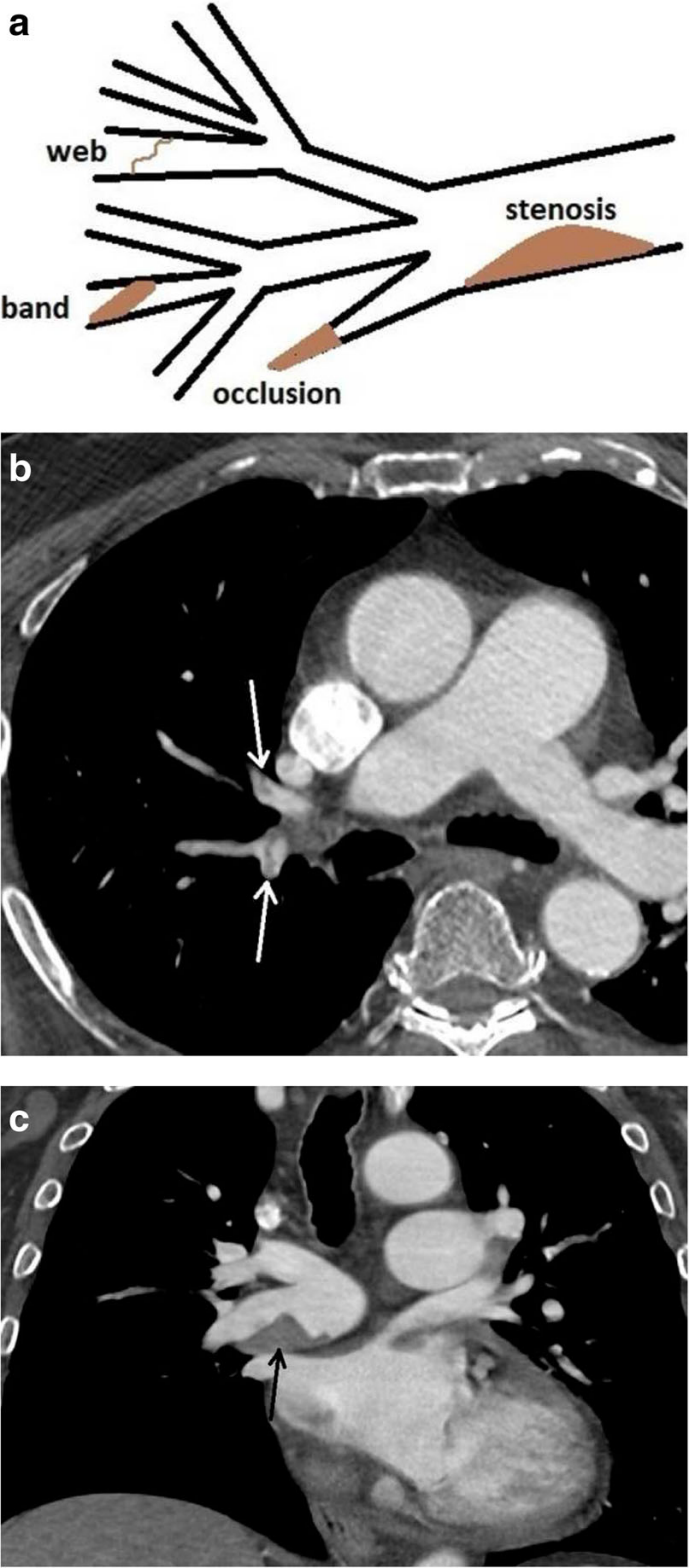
Configurations in CTEPH. a Figure demonstrating findings of CTEPH including stenosis, web, band, and occlusion. b CTA axial image of web/band (white arrows). c CTA axial image demonstrates eccentric thrombus narrowing the lumen of the right main pulmonary artery (black arrow)
